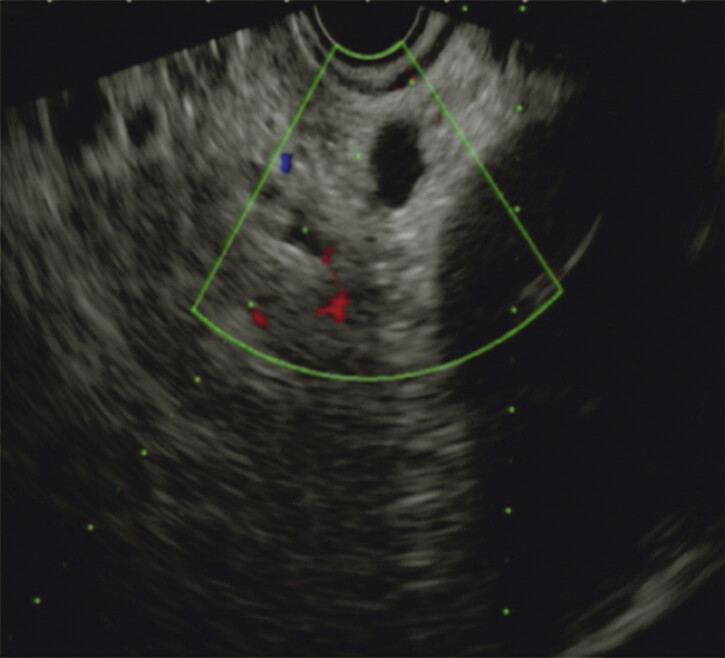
Endoscopic ultrasound image showing an enlarged lymph node in the hepatogastric space.
